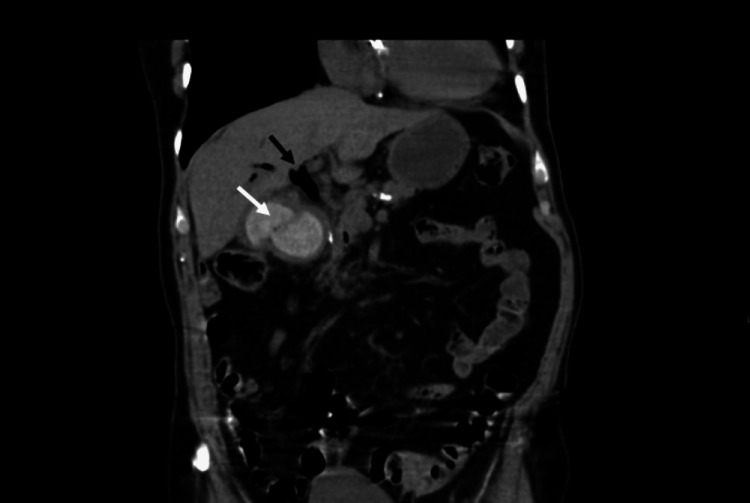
Coronal CT showing gallstone within the lumen of the duodenum (white arrow) and also note gas within the common bile duct (black arrow).
